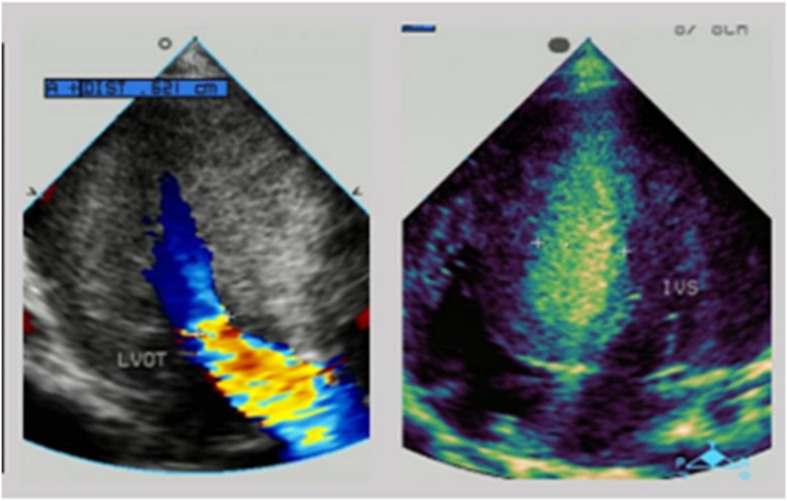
The echocardiogram of patient 1: substantial left ventricular hypertrophy with a maximal thickness in the posterior wall of the left ventricle of 10 mm and a ventricular septal thickness of 15 mm, and obstruction in the left ventricular outflow tract.
